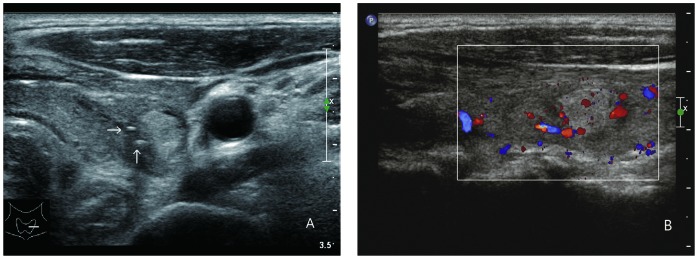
Repeat ultrasound examination six months after the initiation of anti-tuberculosis medication. (A) Significant shrinkage and echo enhancements can be observed. The nodule is solid and well-defined, with two tiny calcifications inside (white arrows). (B) Color Doppler examination revealing punctate and strip-shaped blood flow around and inside the nodule.
